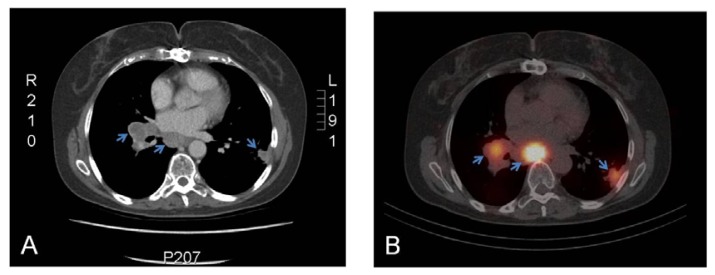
Images of a patient with metastatic RCC. Conventional CT (A) and ^111^In-girentuximab immunoSPECT (B) images of a patient with metastatic ccRCC. Clear and preferential uptake of the radiolabeled antibody was observed in mediastinal and pleural lesions (arrows). The patient was enrolled in the phase II ^177^Lu-girentuximab RIT trial.
